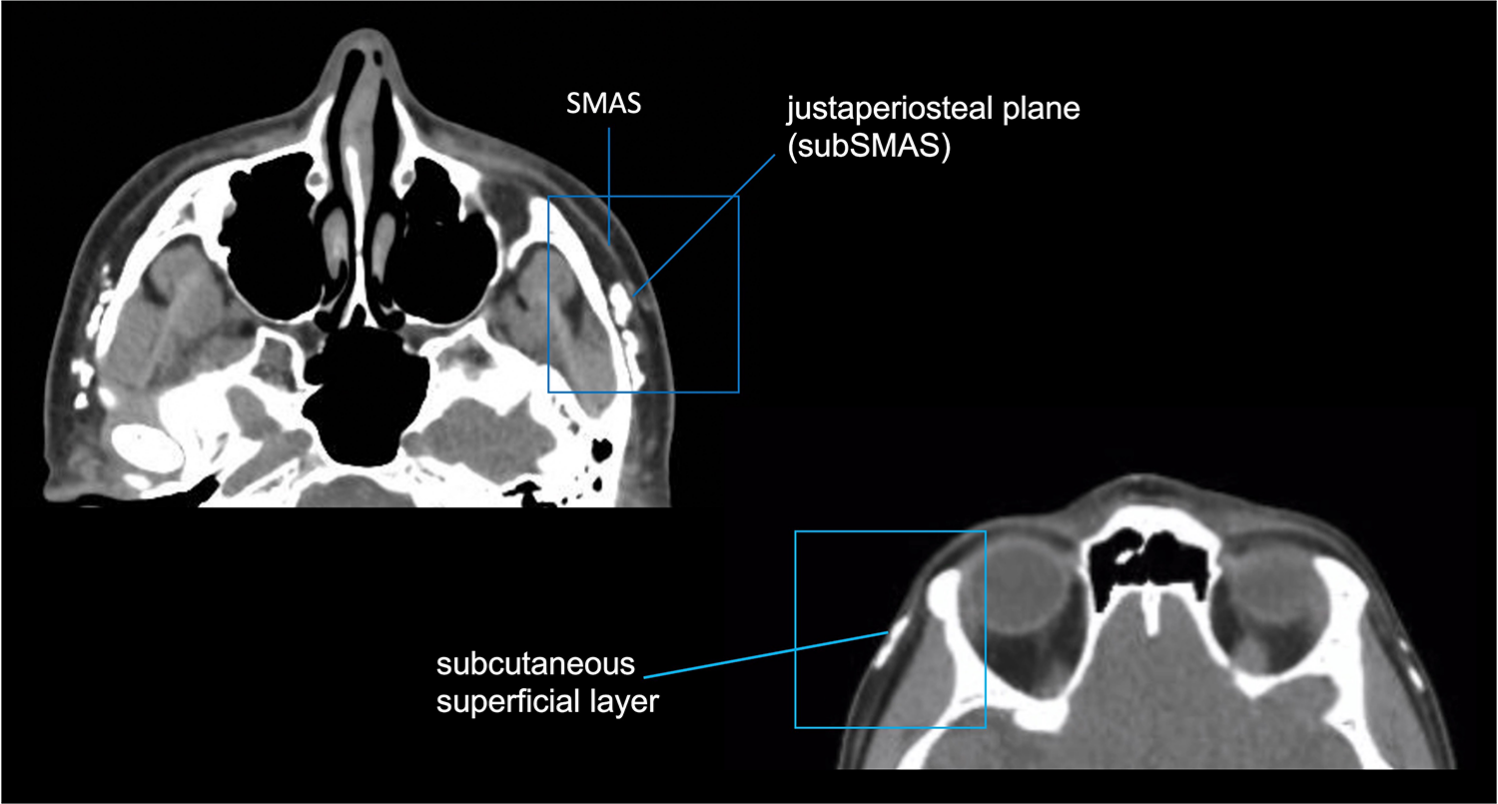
Face CT scan showing CaHa injection in the zygomatic arch in the subSMAS plane (justaperiosteal) and in the temporal region in the subcutaneous plane
